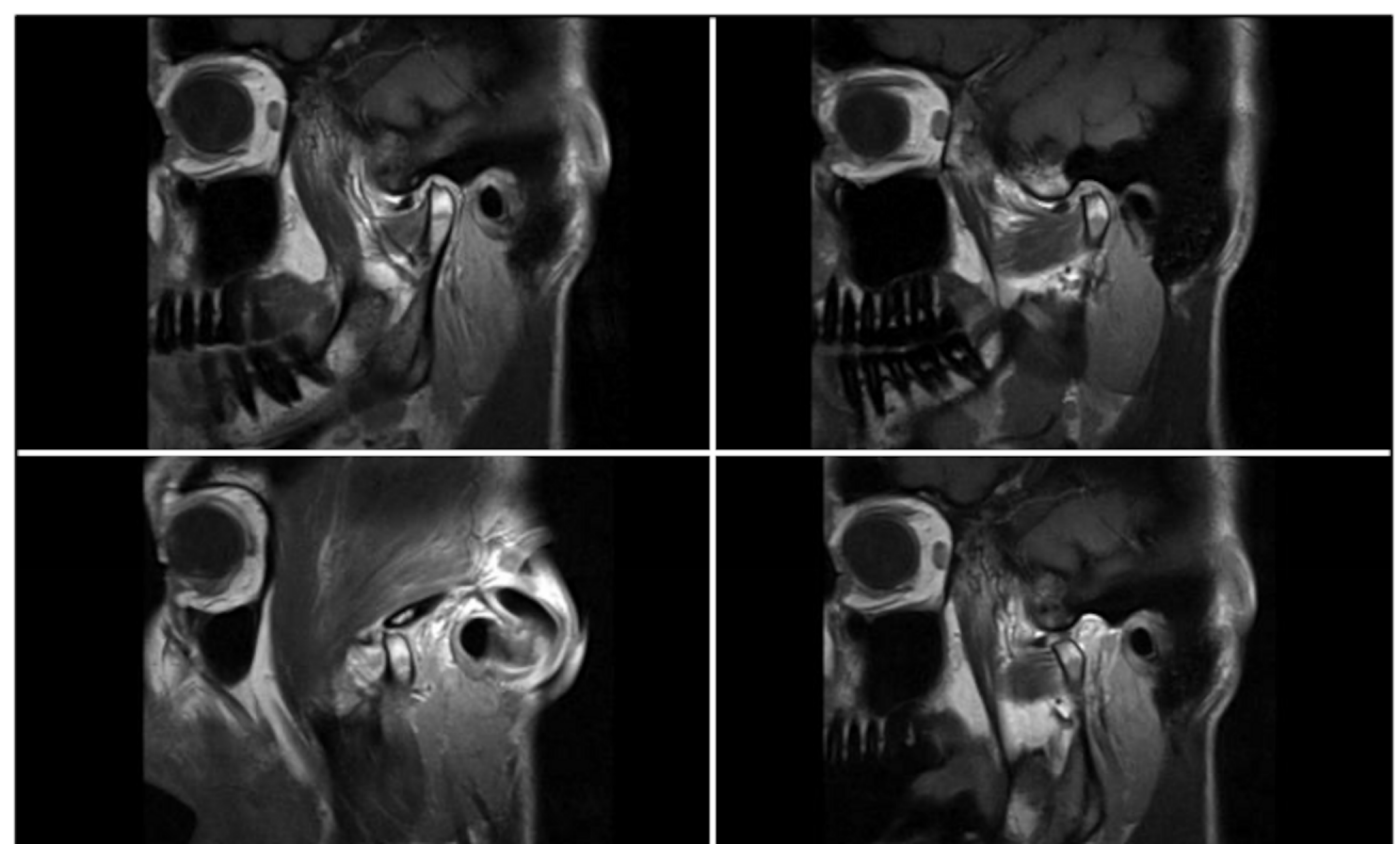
Representative sagittal T1-weighted MRI sections. The upper images display the TMJ in medial (right) and lateral (left) view respectively with the mouth closed. The lower images show the same medial (right) and lateral (left) views with the mouth open. Degenerative changes in the condylar head, as well as disc displacement are visible
